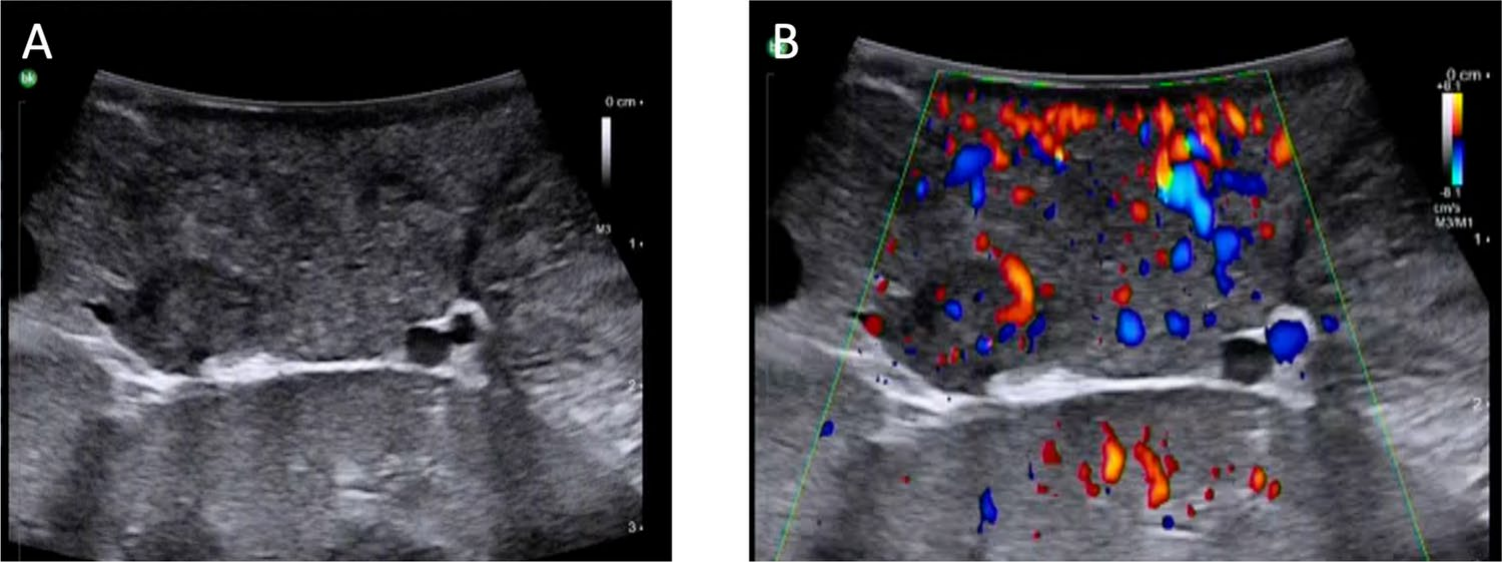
Intraoperative ultrasound (A) and colour-coded duplex sonography (B) during robotic resection of a well-differentiated insulinoma (arrows) in the pancreatic tail
